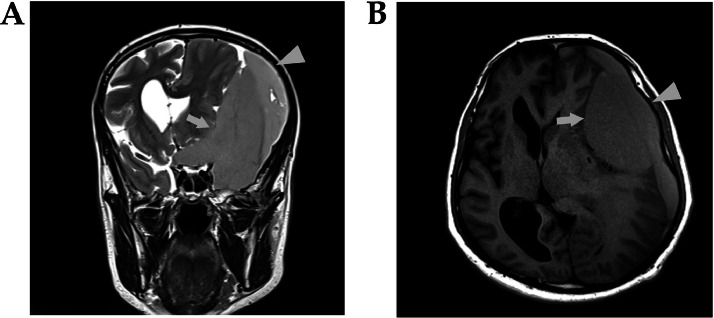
The brain MRI images demonstrate (A) A T2-weighted coronal section and (B) A T1-weighted axial section, revealing an intermediate signal collection on the left side comprising 2 components with varying gray levels. The “arrowhead” highlights the subdural collection, and the “ arrow” marks the cystic collection extending into the suprasellar region.
